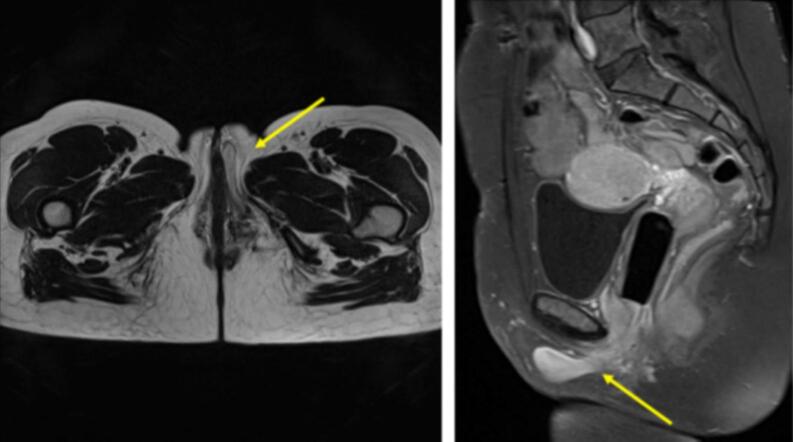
Axial T2 and sagittal T1 pelvic MRI after gadolinium injection and fat signal saturation: tumoral remnant of the labia majora adjacent to the left pelvic floor with the same characteristics as the initial tumor: T2 hyposignal and intense enhancement after gadolinium injection.
